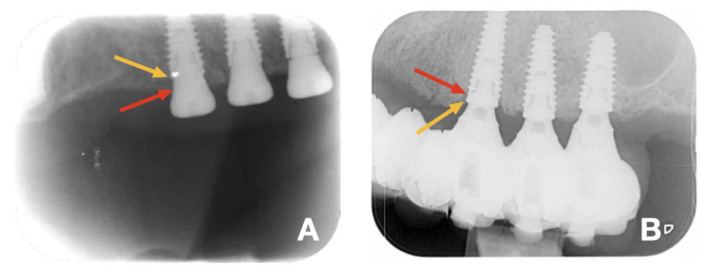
Radiograph obtained at the day of implant placement (A), and after 3–5 years of function (B). The yellow arrow indicates the reference point located at implant–abutment interface; the red arrow indicates the bone-to-implant contact level.
